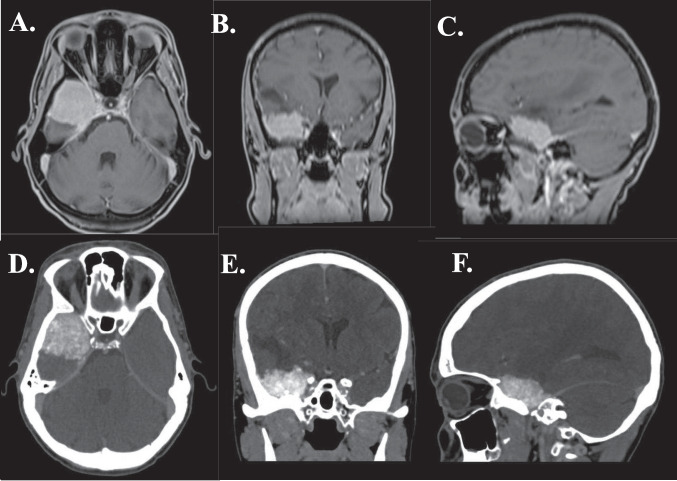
ABC: MRI scan (T1W sequence) of patient with medial sphenoid wing meningioma with optic nerve involvement. DEF: CT scan of patient with medial sphenoid wing meningioma, compressing the optic nerve
